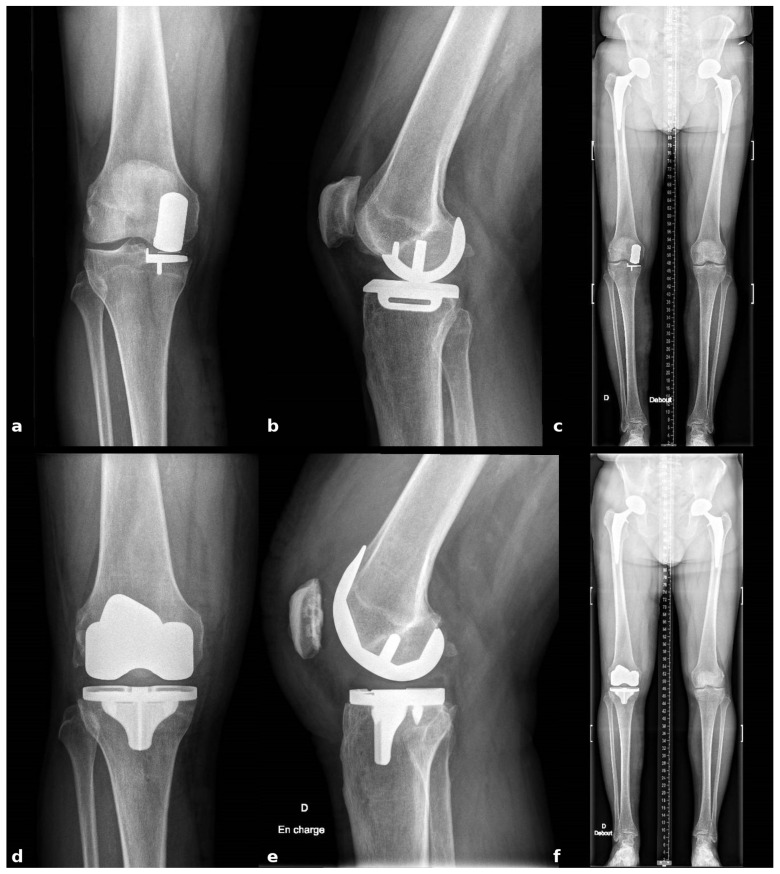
Radiographic comparison of preoperative and postoperative component positioning and alignment (a) Preop AP view; (b) Preop Lateral view; (c) Preop Long-leg alignment view; (d) Postop AP view; (e) Postop Lateral view; (f) Postop Long-leg alignment view.
